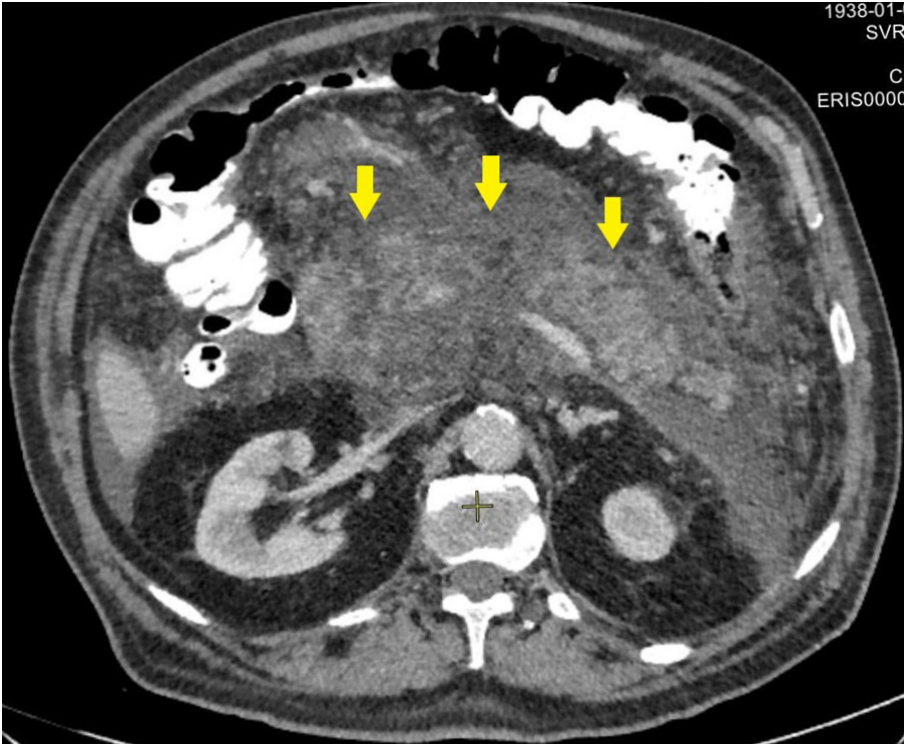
CT image of necrotizing pancreatitis in patients, who failed in endoscopic transpapillary stent placement of the main pancreatic duct (peripancreatic infiltrate is indicated by arrows), 72 h after a failed attempt.
